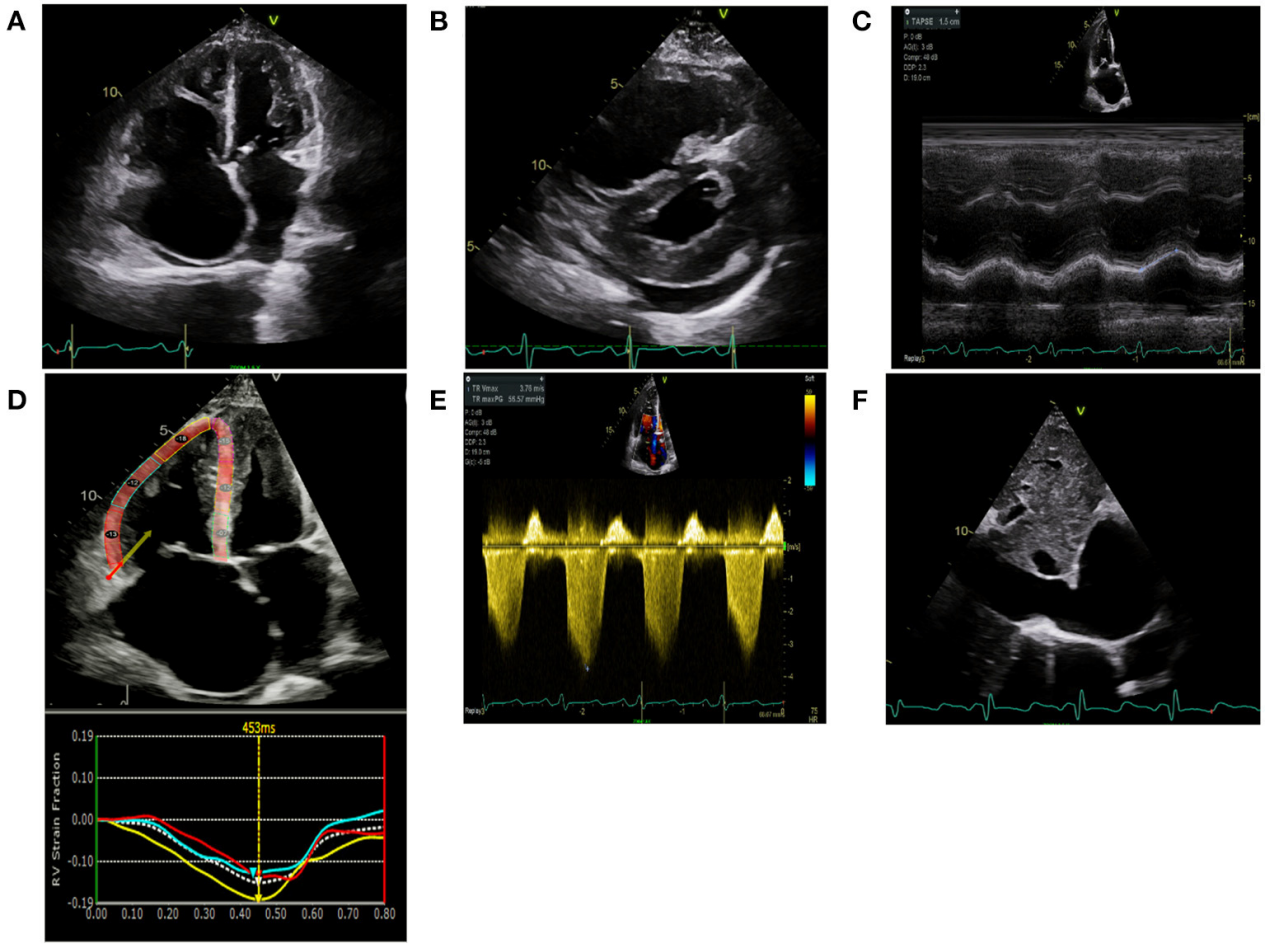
Echocardiographic images are shown in a scleroderma patient with severe pulmonary hypertension on stable therapies. (A) Apical 4 chamber view demonstrates severe right atrial enlargement with bowing of the interatrial septum from right to left suggestive of elevated right atrial pressures. The right ventricle is severely dilated and hypertrophied with a prominent moderator band. The left ventricle is hypertrophied and small. (B) Parasternal short-axis is shown in the same patient with marked RV enlargement and evidence of RV pressure overload distorting the normal circular short-axis geometry of the LV. There is a small posterior pericardial effusion present. (C) Tricuspid annular plane systolic excursion (TAPSE) utilizes M-mode techniques to measure the longitudinal motion of the basal right ventricular wall segment during systole as an estimate of right ventricular systolic function. TAPSE is mildly reduced at 1.5 cm (normal >1.6 cm) however fractional area change was 24% (moderate-severely reduced). (D) Right Ventricular Longitudinal Systolic Strain (RVLSS) is a recent echocardiographic advancement based on ultrasound-myocardial tissue interactions. Each segment of the RV in this example corresponds with a strain curve with the white dotted line representing an average of the segmental strain for the regional curves in this view. Regional RV free wall strain is reduced in the basal and midventricular wall segments with less reduction in the apical segment. Global strain is an average of the three RV free wall segments and is −14.3%. (E) Right Ventricular Systolic Pressure utilizes the peak tricuspid velocity to calculate the peak right ventricular systolic pressure using the modified Bernoulli equation. RVSP= [peak gradient (mmHg) = right atrial pressure + (4 × Peak velocity 2)]. In this example, RVSP = 57 mmHg + 15 mmHg = 72 mmHg. (F) Right atrial pressures are estimated from the IVC diameter made in subcostal view at end-expiration. In this example, the IVC is severely dilated at 3.2 cm with minimal respiratory variation suggestive of markedly elevated right atrial pressure of 15 mmHg.
